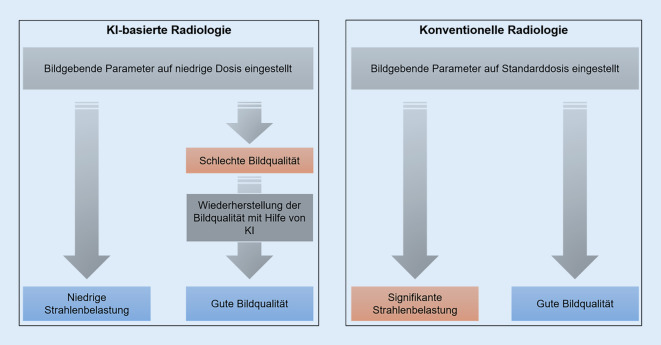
Im Folgenden werden einige Studien besprochen, in denen KI-Algorithmen entwickelt und eingesetzt wurden, um Bilder zu entrauschen, die unter Niedrigdosisbedingungen aufgenommen wurden, z. B. bei niedrigem kVp, niedrigem mAs oder niedriger Aktivität, und ihre Qualität so zu verbessern, dass sie dem Standard von Normaldosisbildern entspricht. Der Inhalt ist in 5 Abschnitte gegliedert, die den häufig verwendeten Bildgebungsmodalitäten CT, PET, Einzelphotonen-Emissions-Computertomographie (SPECT), Angiographie und Fluoroskopie entsprechen.
